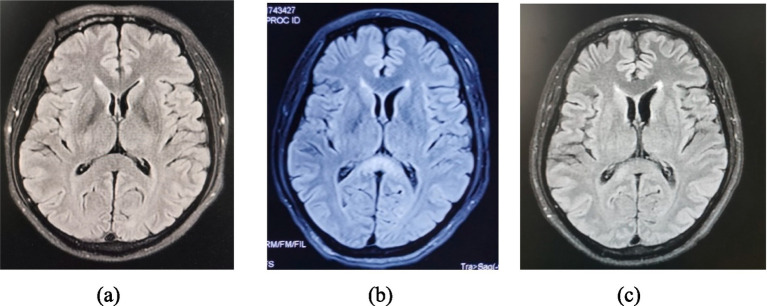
MRI brain FLAIR showed a hyperintense signal in the splenium on the 20 days (A), 37 days (B) and 5.5 months (C) of illness, with obvious improvement of lesions splenium.
